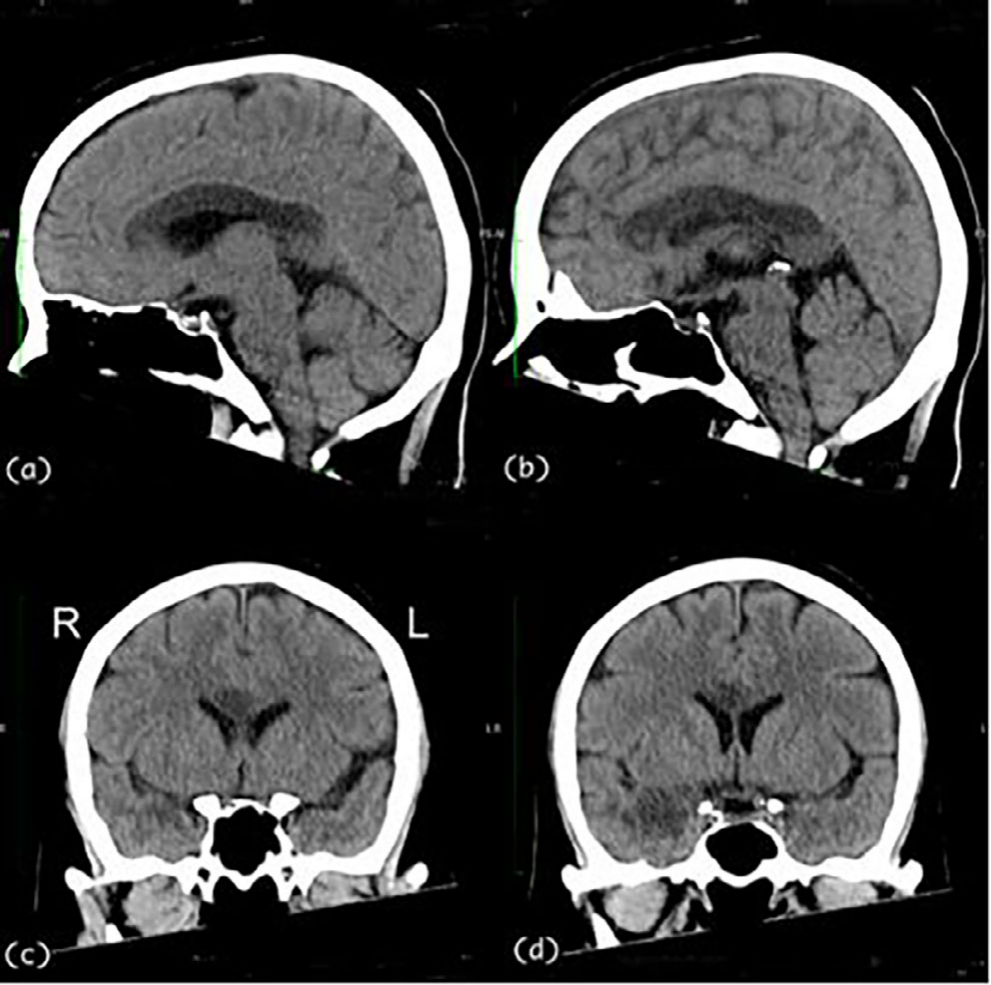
Sagittal [(a) & (b)] and coronal [(c) & (d)] views of the CT Brain. The CT images demonstrate an extensive hypodensity from genu to the splenium of the corpus callosum and the right temporal lobe. CT, computed tomography.
